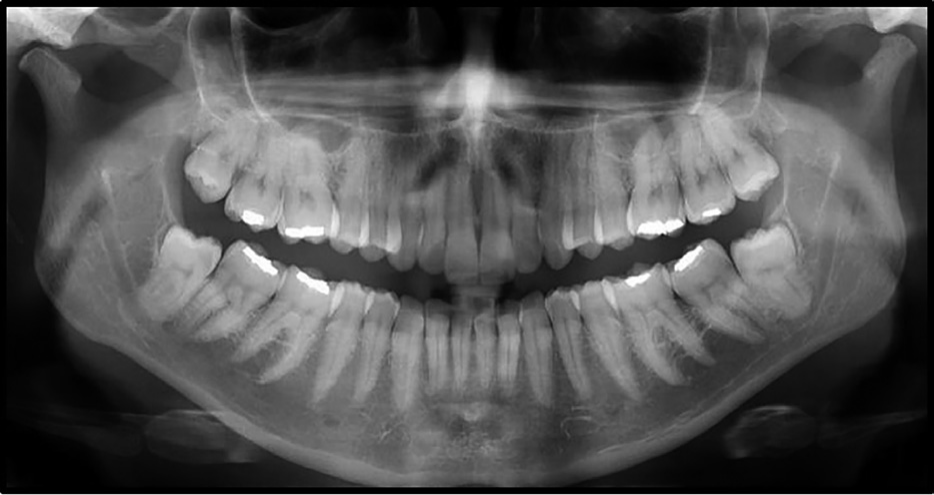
Panoramic radiograph prior to procedure for removal of teeth 38 and 48 presented by the patient in the emergency ward.
